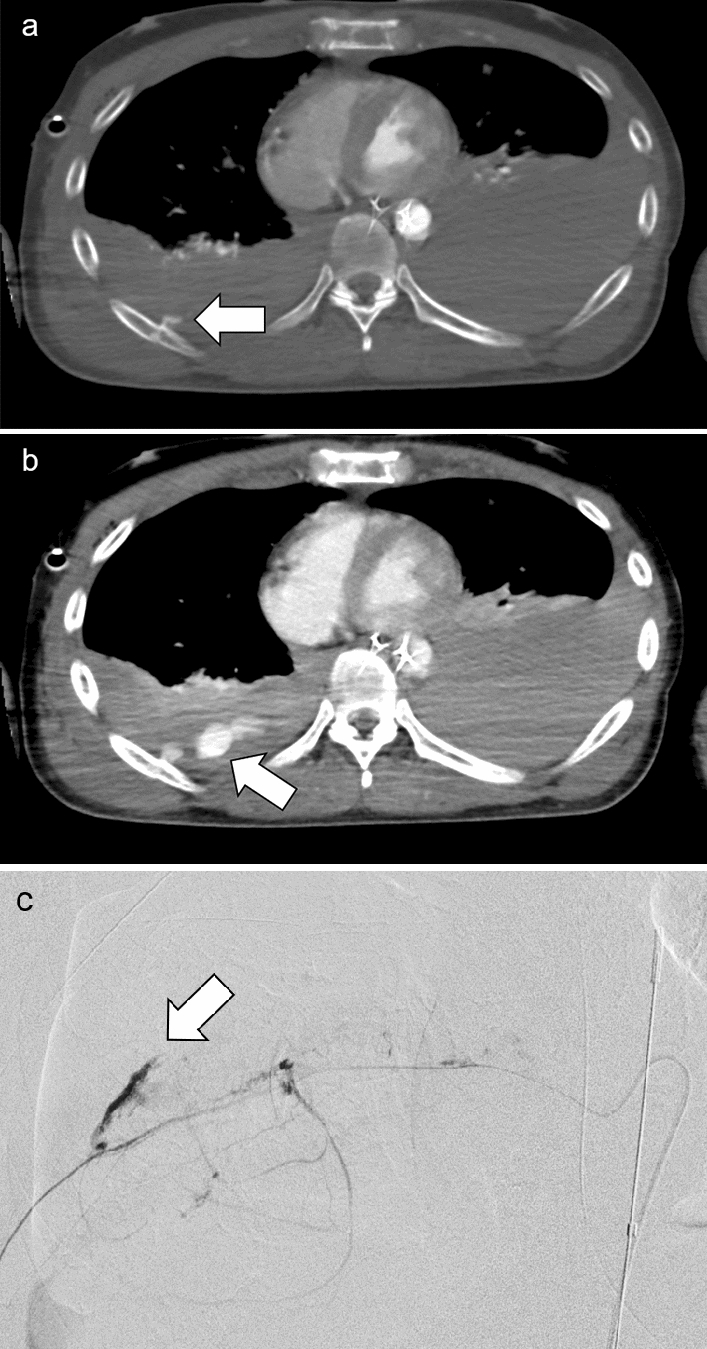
Thoracic contrast-enhancement computed tomography (a: arterial phase, b: venous phase) with Zone I resuscitative endovascular balloon occlusion of the aorta under temporally deflated status after total inflation in the patient with multiple traumas showing extravasation (a and b, arrow) from the right intercostal artery, which was confirmed by angiography (c, arrow). The bleeding was treated by transcatheter arterial embolization
